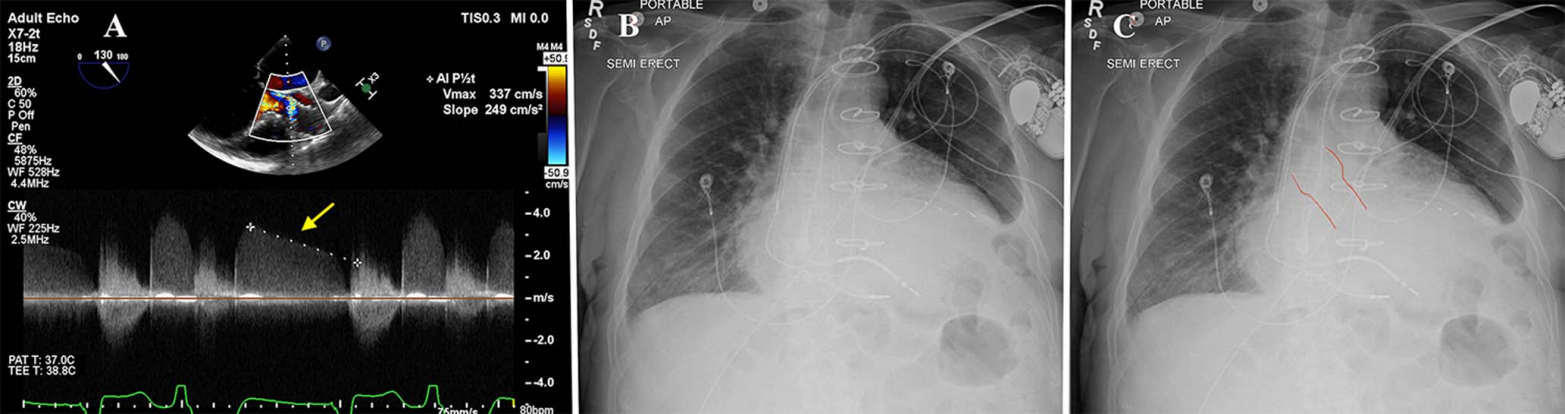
(A) Transesophageal Doppler with dense regurgitation signal and markedly reduced pressure half-time suggestive of severe CoreValve (Medtronic) insufficiency. (B) Anteroposterior chest x-ray before the CoreValve implantation, showing cardiomegaly and pulmonary congestion. (C) Anteroposterior chest-x-ray after the CoreValve implantation with the CoreValve outlined.
